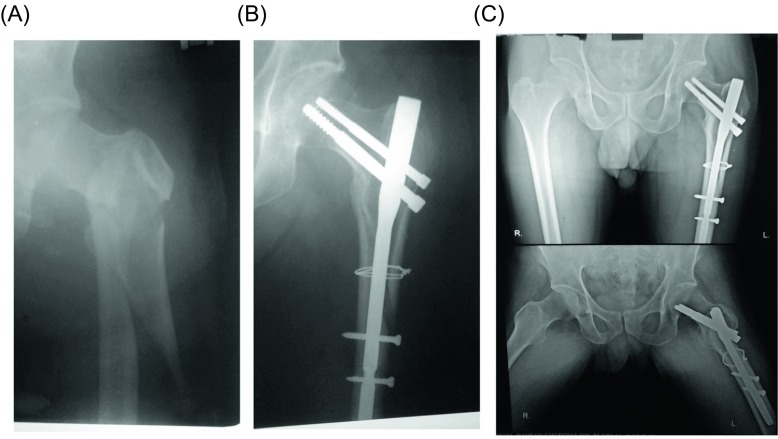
A 70-year-old female. (A) The initial preoperative radiographs. (B) Radiograph six weeks postoperatively with cerclage wire. (C) Anteroposterior radiograph at eight months postoperatively demonstrating the fracture healing.
